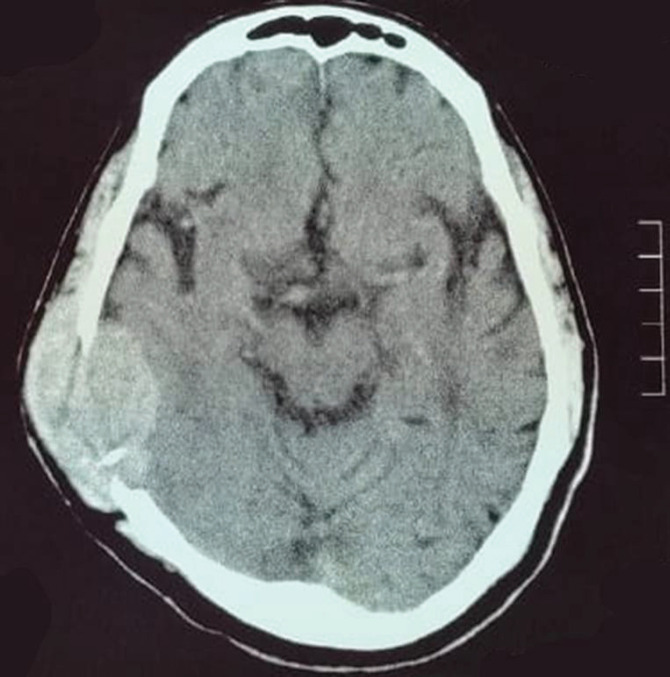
CT brain axial slice shows soft tissue mass within the right temporoparietal bone causing bone destruction, and compression of adjacent cortical sulci.
